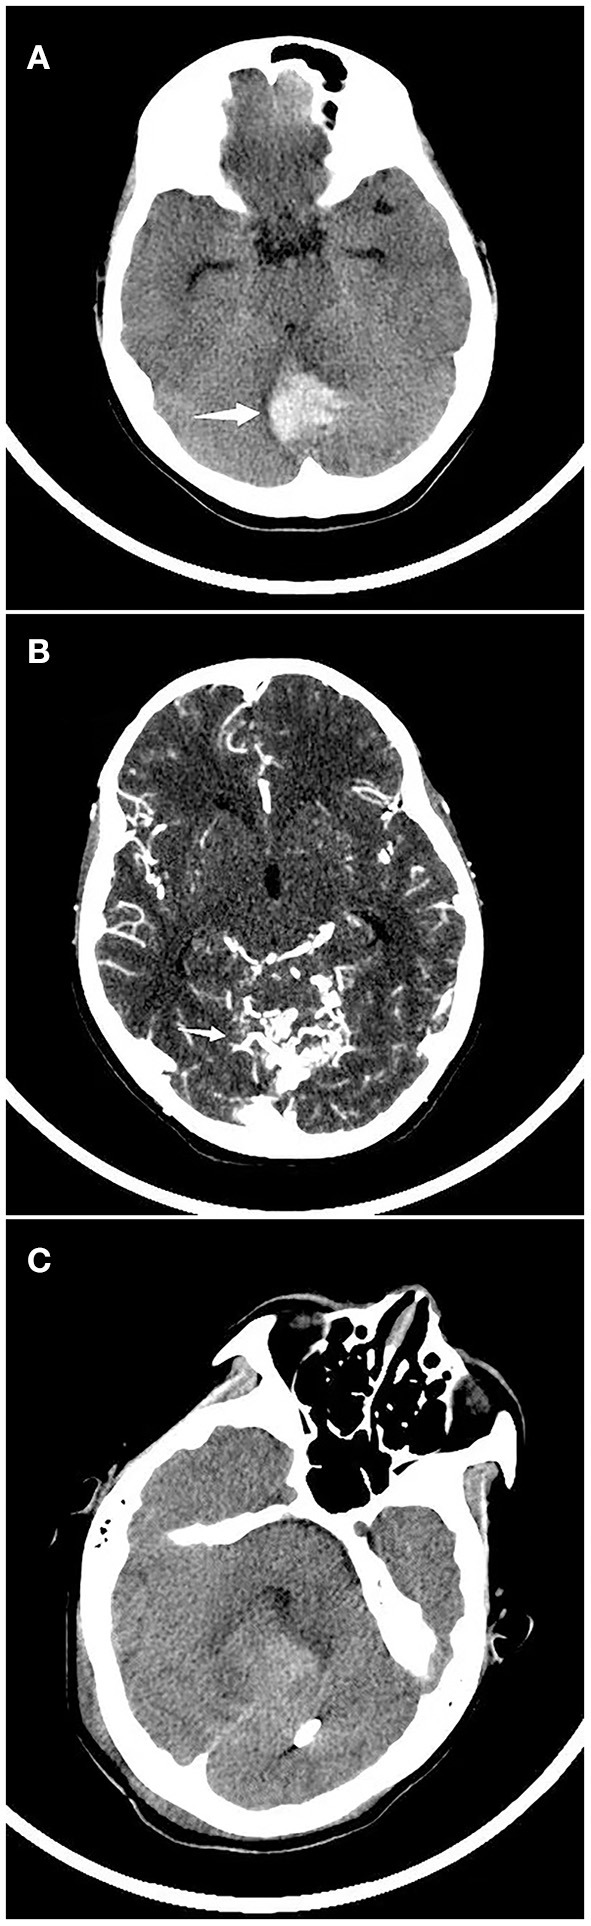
Continuous CT imaging of a child with spontaneous cerebral hemorrhage. (A) A child was admitted to the hospital due to vomiting and dizziness, and a head CT examination showed that that cerebellar hemorrhage. (B) CT angiography suggested arteriovenous malformations. (C) Head CT was reviewed 2 weeks after arteriovenous malformation embolization.
